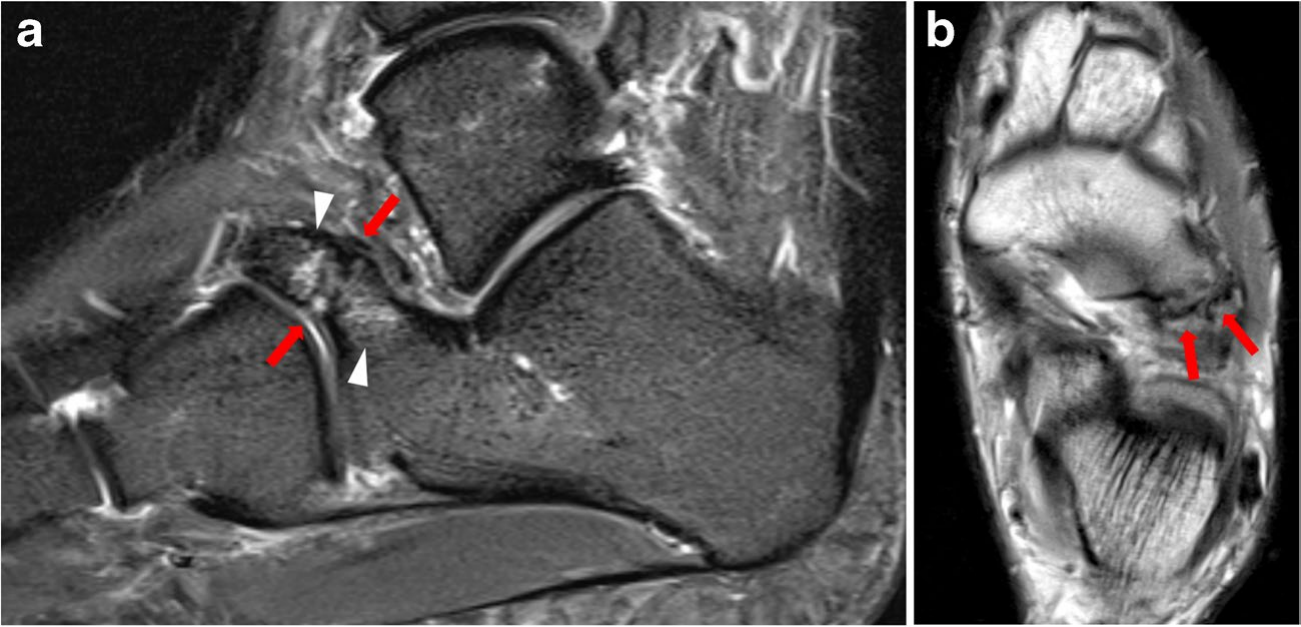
23-year-old female patient admitted with pain of the left foot and calcaneonavicular coalition, correctly diagnosed by all three readers. Calcaneonavicular coalition (arrows) with adjacent osseous marrow edema (arrowheads) is seen on sagittal STIR image and axial T1-weighted image (a, b)
